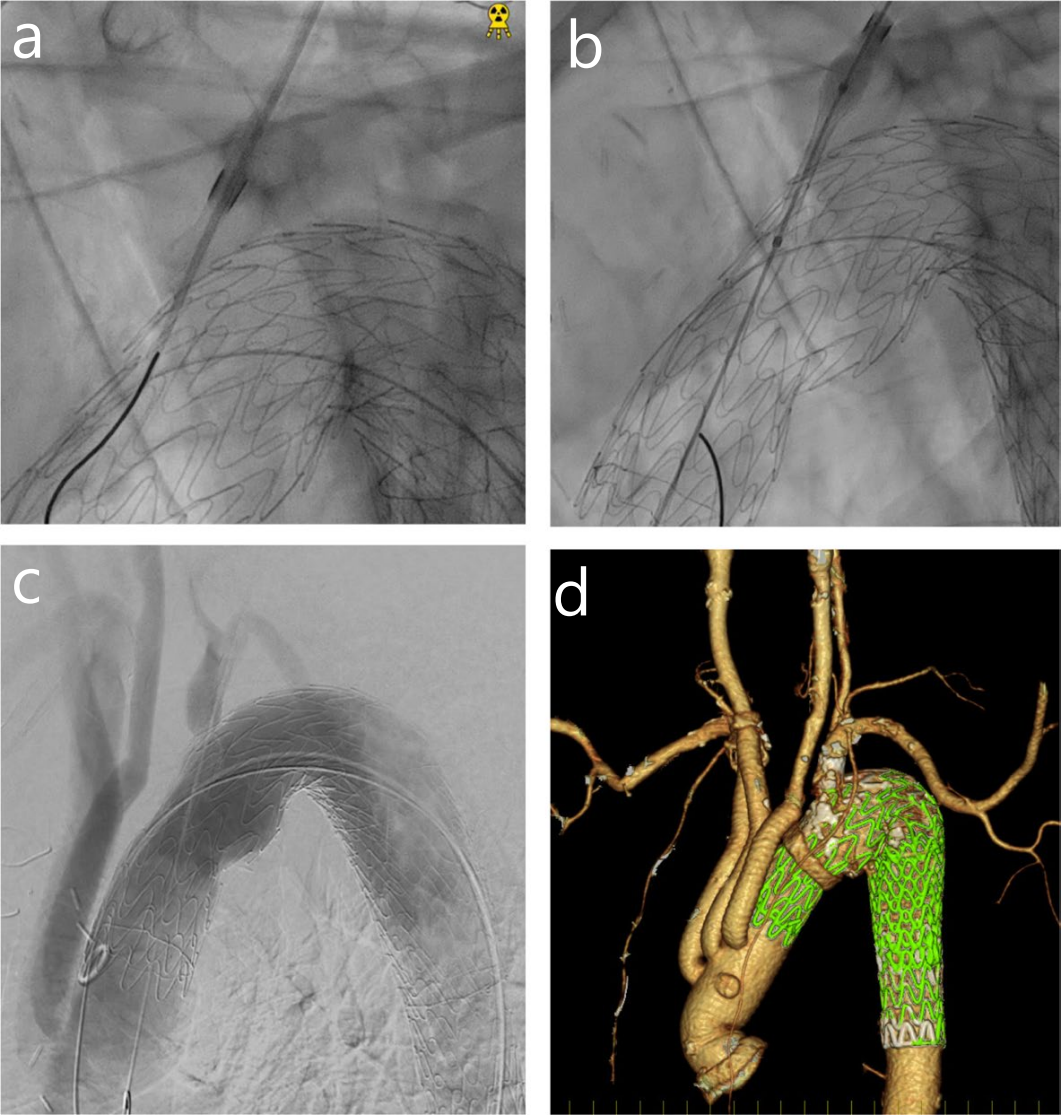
a An 18-guage percutaneous transhepatic gallbladder drainage needle advanced into the stent graft. b An 11–29-mm Viabahn endoprosthesis advanced into the stent graft, and the puncture hole was deployed. c Post-surgery angiography. d Postoperative three-dimensional computed tomography image
